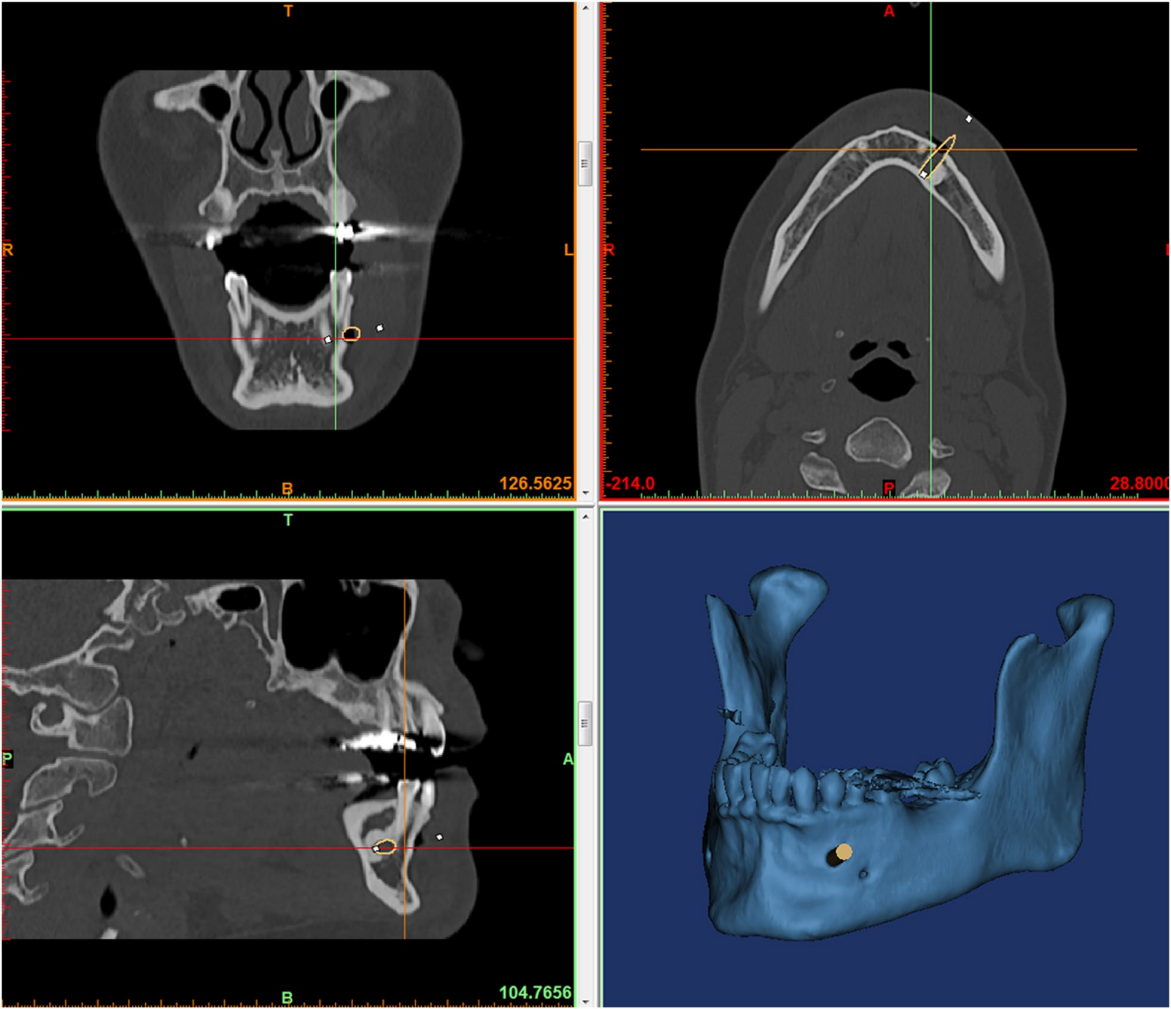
Screenshot of planning the biopsy channel according to CT data
